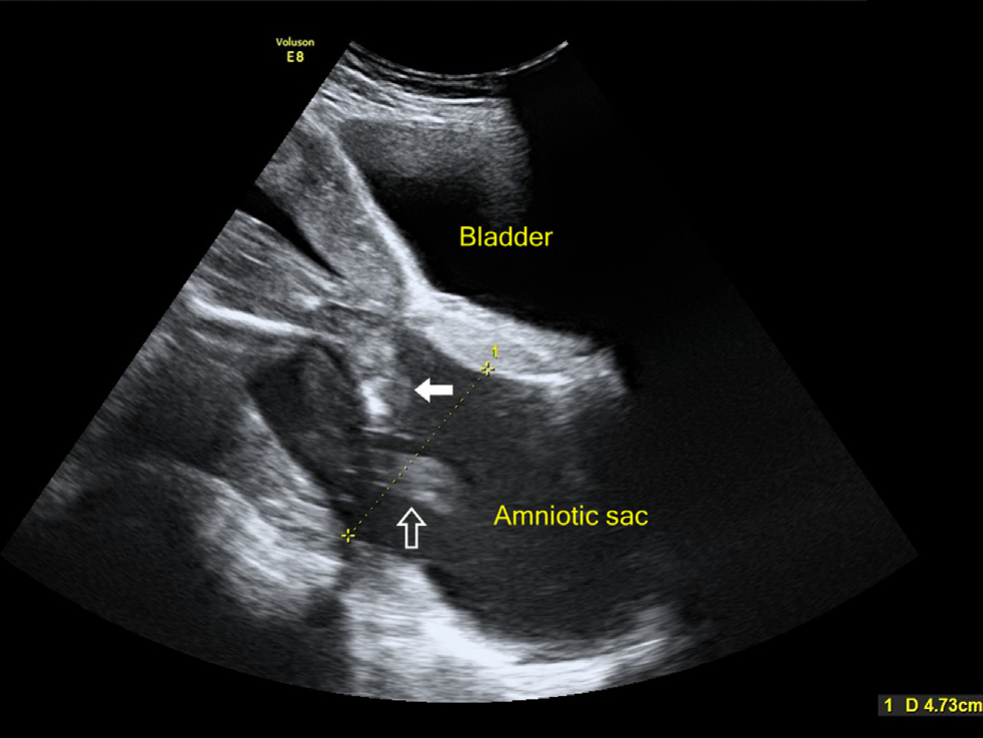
Abdominal ultrasonography revealed the amniotic sac bulging through the cervix, creating a classic “hourglass appearance,” with the fetal legs (Fig 2, arrow) and umbilical cord (Fig 2, hollow arrow) protruding. The cervix dilated to approximately 4.73 cm.
